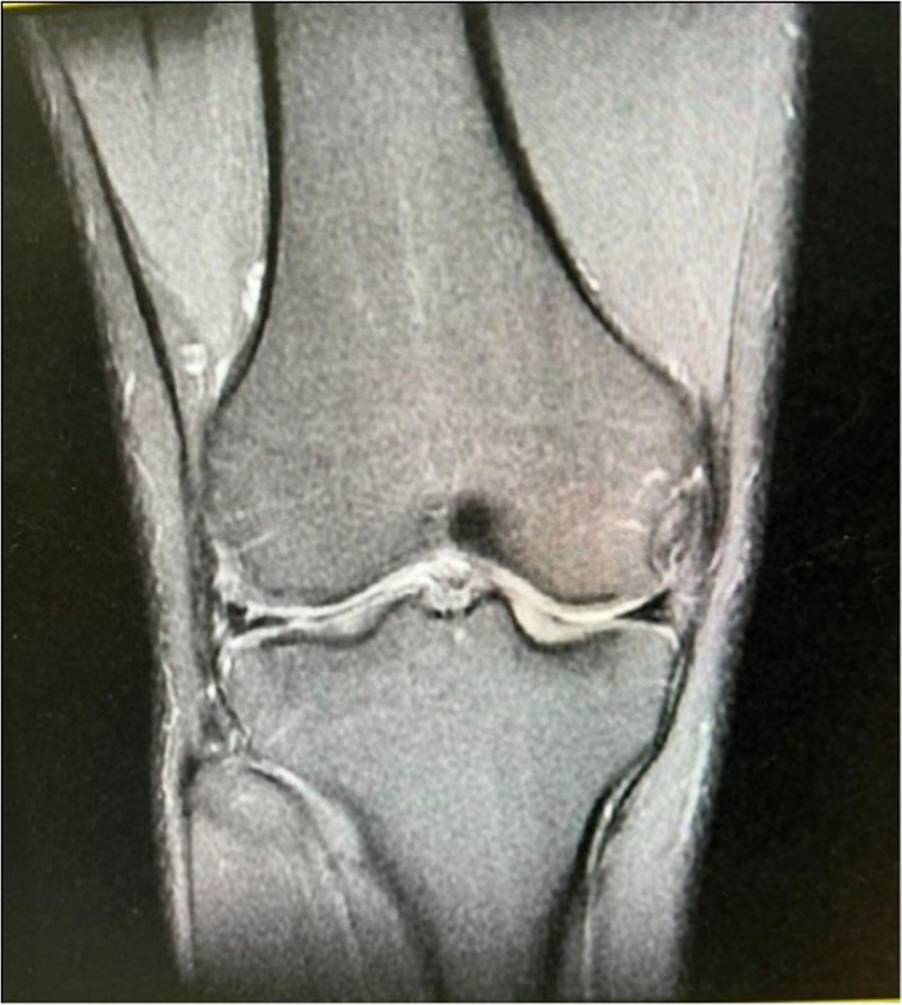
Knee magnetic resonance imaging of patients with mid‐substance medial collateral ligament ligament injury.
